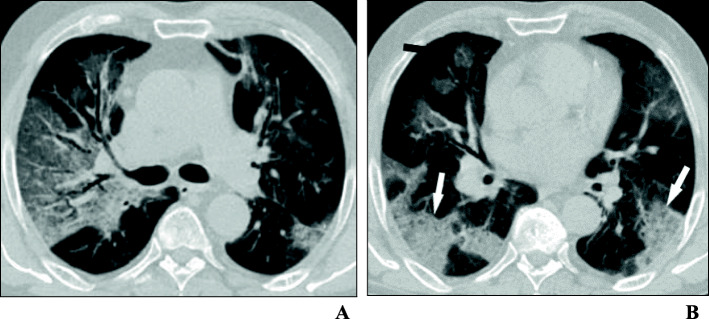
A 66-year-old woman who presented with fever, productive cough, and dyspnea of 10 days duration. Her PaO_2_/FiO_2_ ratio was 250 mmHg. Axial thin-section unenhanced CT images shows a a crazy-paving pattern with traction bronchiectasis and b ground-glass opacities with round configuration (black arrow) and posterior consolidation (white arrows)
